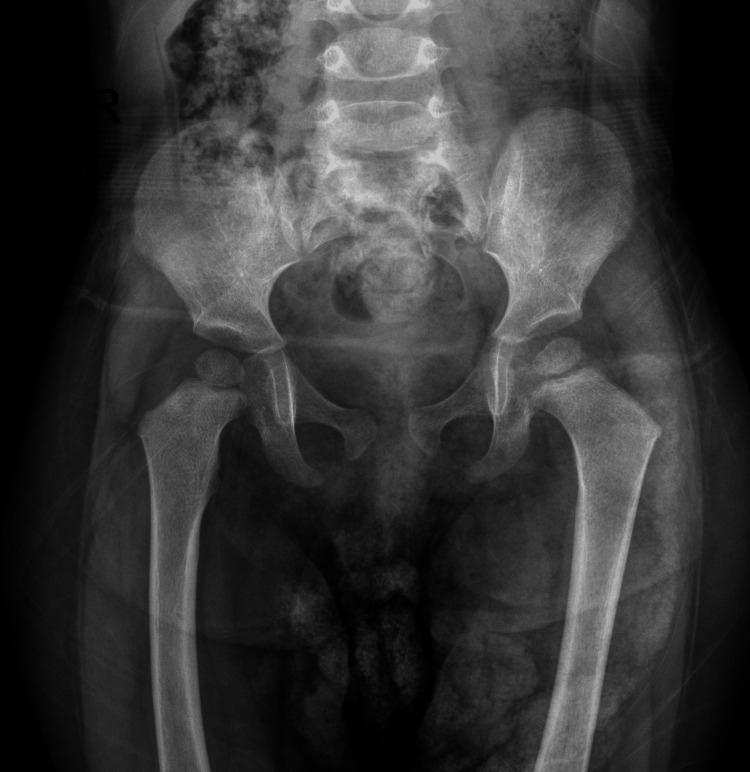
AP pelvis X-ray at presentation
